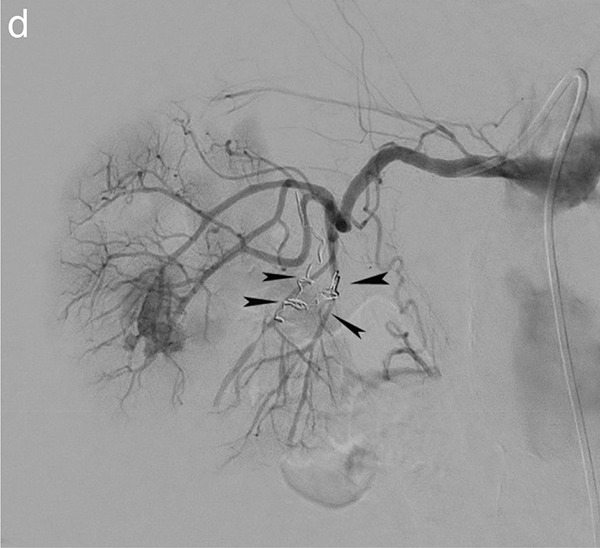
a. Contrast‑enhanced T1‑weighted MR‑image shows a hypovascular mass lesion (arrows) in the right anterior lower pole. b. Unenhanced, reconstructed parasagittal CT‑image during percutaneous cryoablation procedure shows exophytic mass lesion (arrows) and four needles within the mass lesion. c. Procedural retroperitoneal hemorrhage occurred and subsequent emergent selective angiography revealed two bleeding points (arrows) at the lower pole of the right kidney. d. Selective angiography after coil‑embolization (arrowheads) shows complete occlusion of the bleeding renal end branches.
